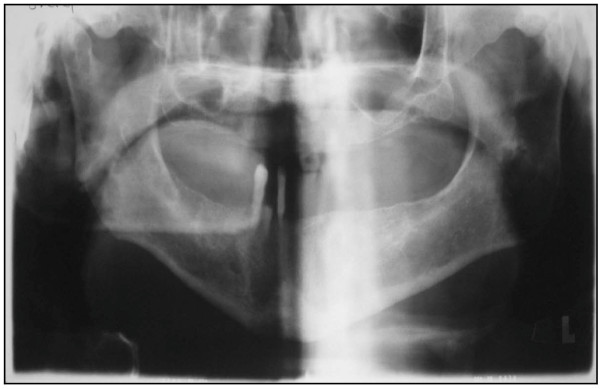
Radiographic findings from the patient described in this case report. Diffuse osteopeny in mandibular and prominence trabeculation of maxilla are present.
